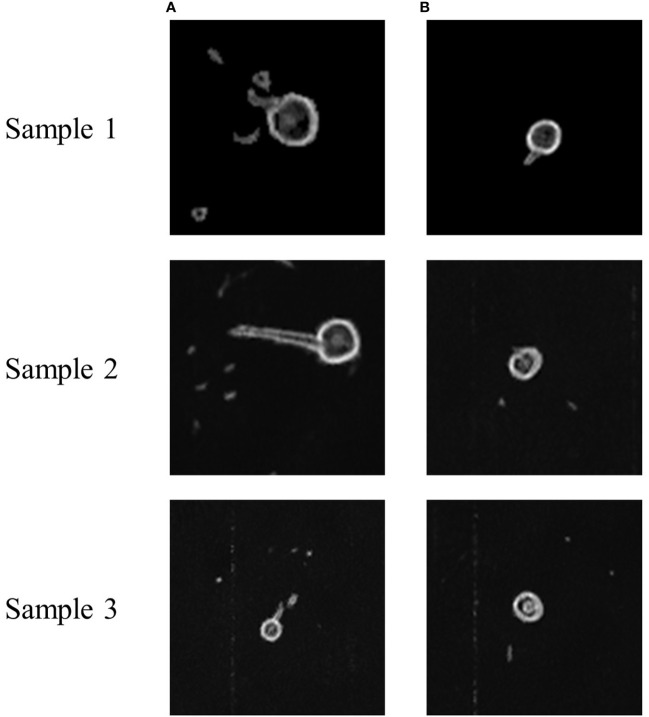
Sample MRI images of healthy and diseased roots of cotton. (A) Healthy cotton root system, (B) Infected cotton root systems. These sample images are from the same location of different root systems.
